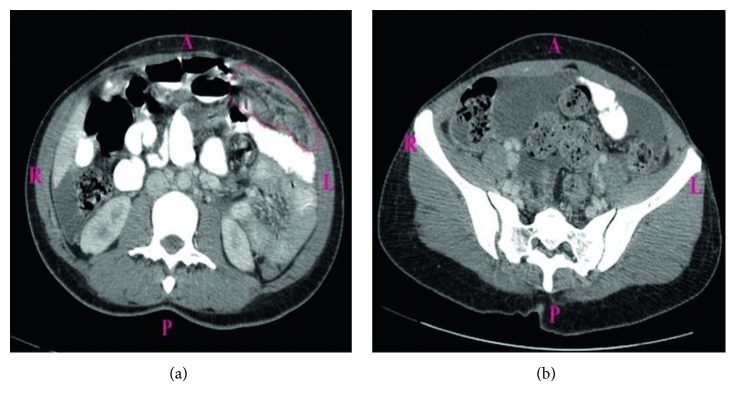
Computed tomography of the abdomen and pelvis with contrast. Diffuse mesenteric enhancement in the left upper quadrant suspicious for carcinomatosis (a) and multiloculated cystic mass adjacent to the right adnexa suspicious for ovarian neoplasm (b).
